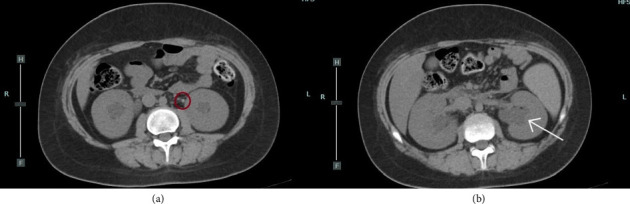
(a) CT scan. The circle indicates the stone located in the left ureter. (b) CT scan. The arrow indicates the dilation of the left pelvi.
